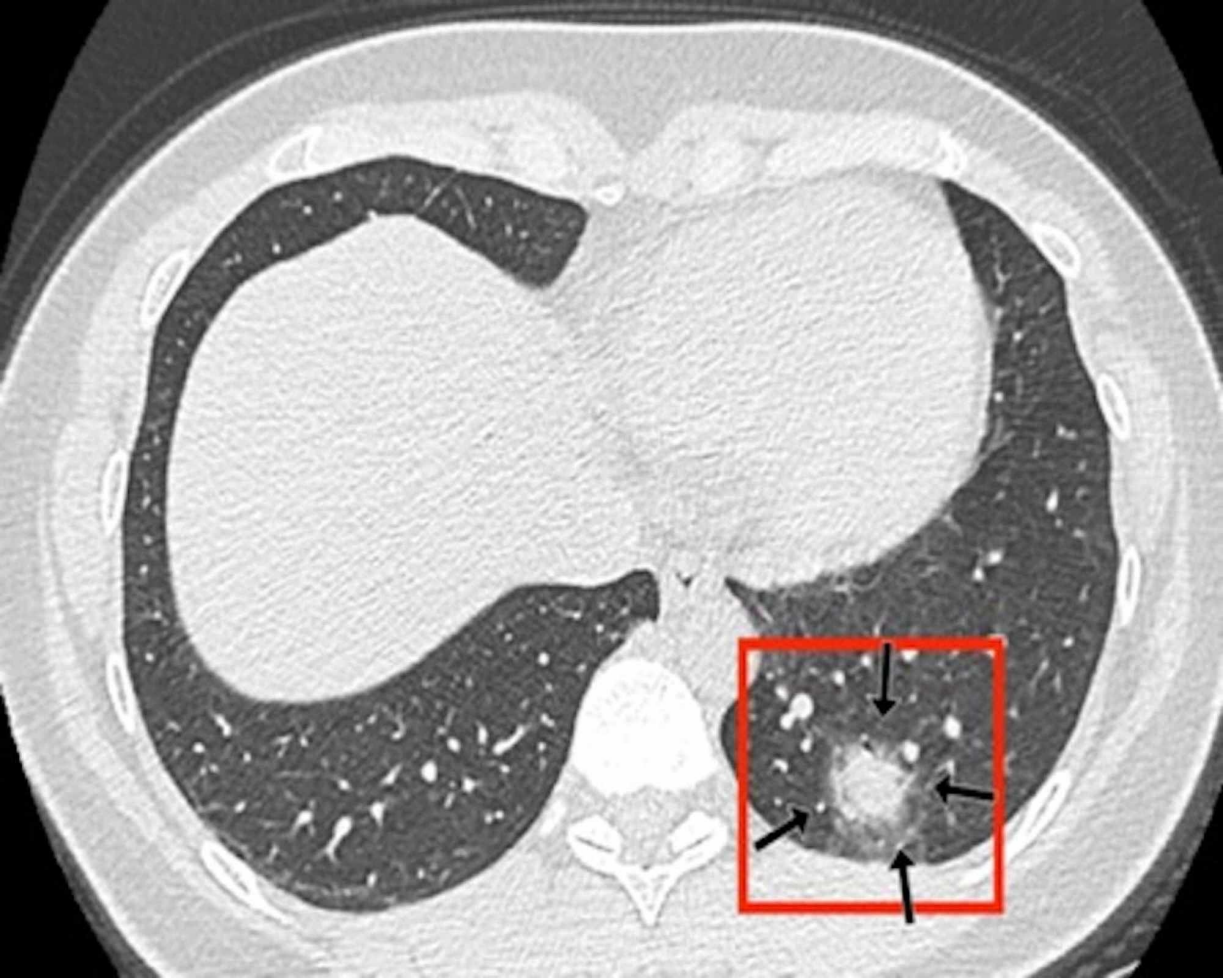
CT halo sign A 27-year-old female patient presented with cough, loin to groin pain, and fever. A CT of the abdomen was performed to look for renal calculi. However, in view of her cough, her chest was also scanned for evidence of COVID-19. An axial non-contrast chest CT image demonstrated an example of a halo sign. There was a discrete focal consolidation within the left lower lobe as outlined in the red box. The nodule showed a peripheral rim of ground-glass opacification, halo sign (black arrows) COVID-19: coronavirus disease 2019; CT: computed tomography
